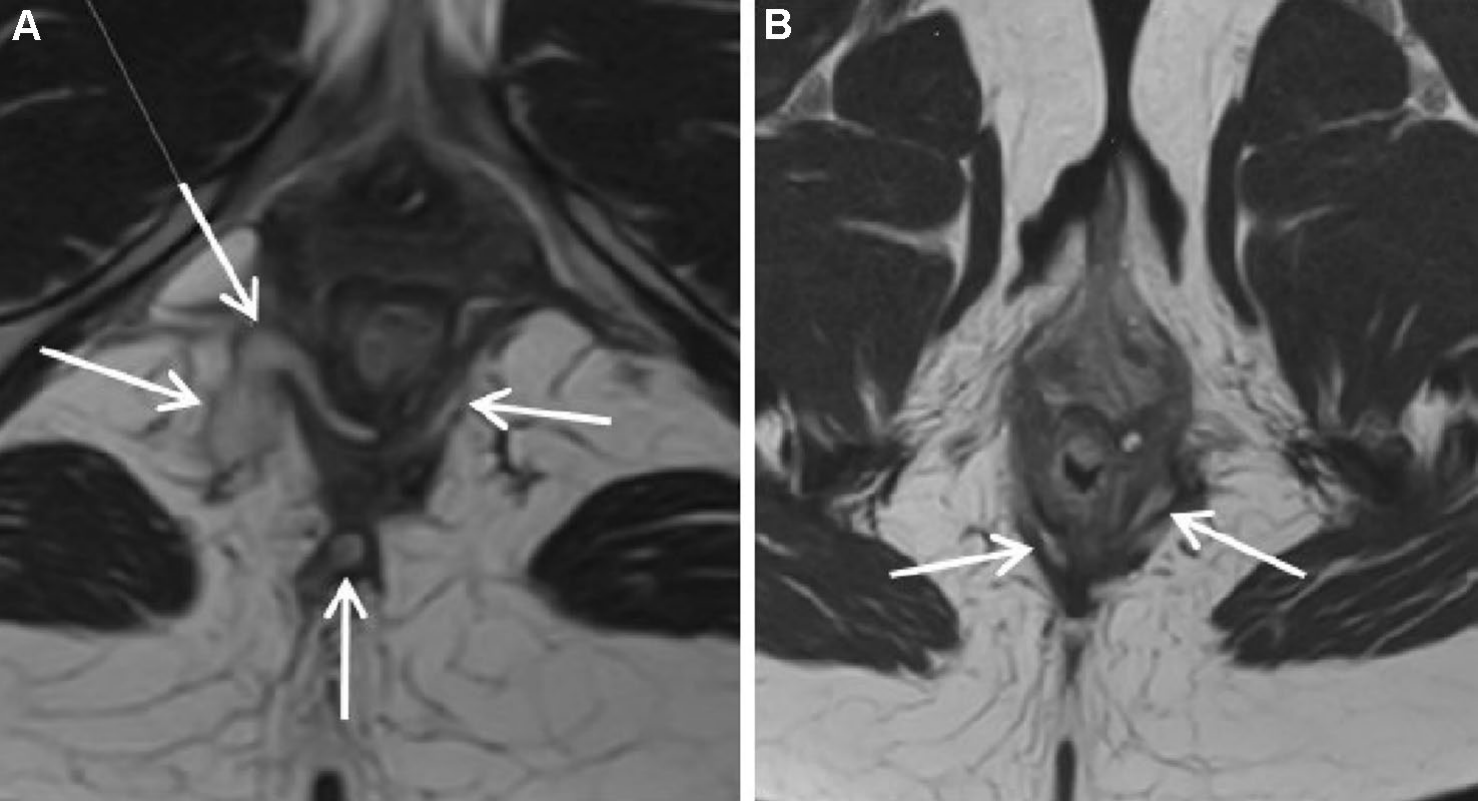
Preoperative and postoperative MRI in a patient with fistula healing, showing incomplete obliteration of the fistula tract: A preoperative MRI (transverse view) of a transsphincteric fistula, showing parts of the fistula tract at both lateral side and in the posterior midline; B postoperative MRI (transverse view) at 6 months shows incomplete obliteration of the fistula tract with remains of the fistula in the intersphincteric plane at the right and left sides. *MRI* magnetic resonance imaging
